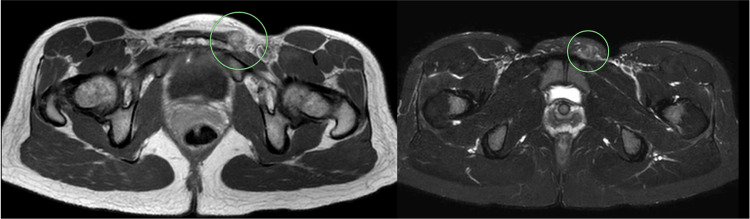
MRI from 16-month follow-up This MRI was performed on the patient approximately 16 months after the original robotic-assisted hernia repair and coincided with her cyclic maximal pain. Note: these images clearly demonstrate inflammation near the left pubic tubercle and the round ligament, which later correlated with endometrial implants on the round ligament. MRI, magnetic resonance imaging.
